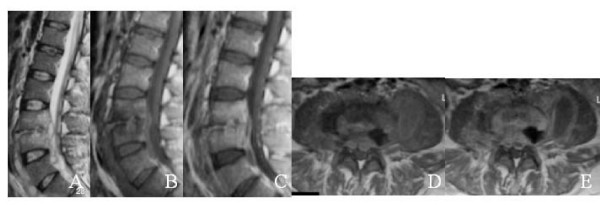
A) T2-weighted image shows increased signal intensity intervertebral disc space with epidural and prevertebral abscess. B) T1-weighted image shows irregularity and destruction of vertebral end plates with iso-to hyperintence signal intensity. C) T1-weighted image after contrast injection shows increased signal intensity intervertebral disc space and the inferior and superior end plates of vertebral bodies. D) T1-weighted axial image shows epidural and paravertebral abscess with iso-to hyperintence signal intensity. E) T1-weighted axial image after contrast injection shows increased signal intensity epidural and paravertebral abscess
